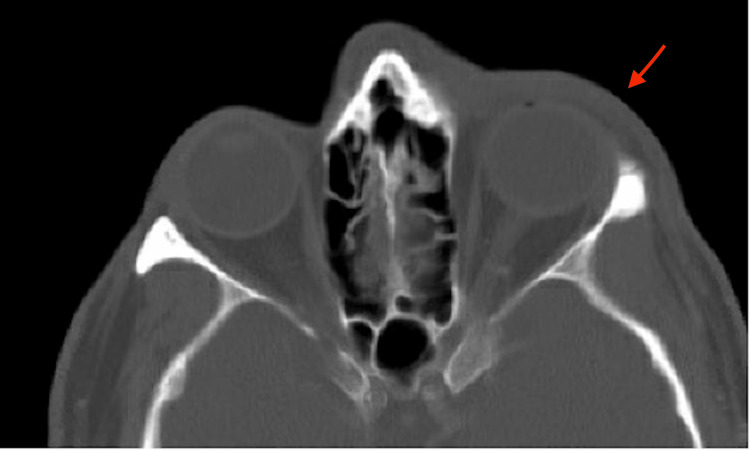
CT scan of the face with contrast indicates left periorbital preseptal cellulitis (red arrow) and left cheek cellulitis
